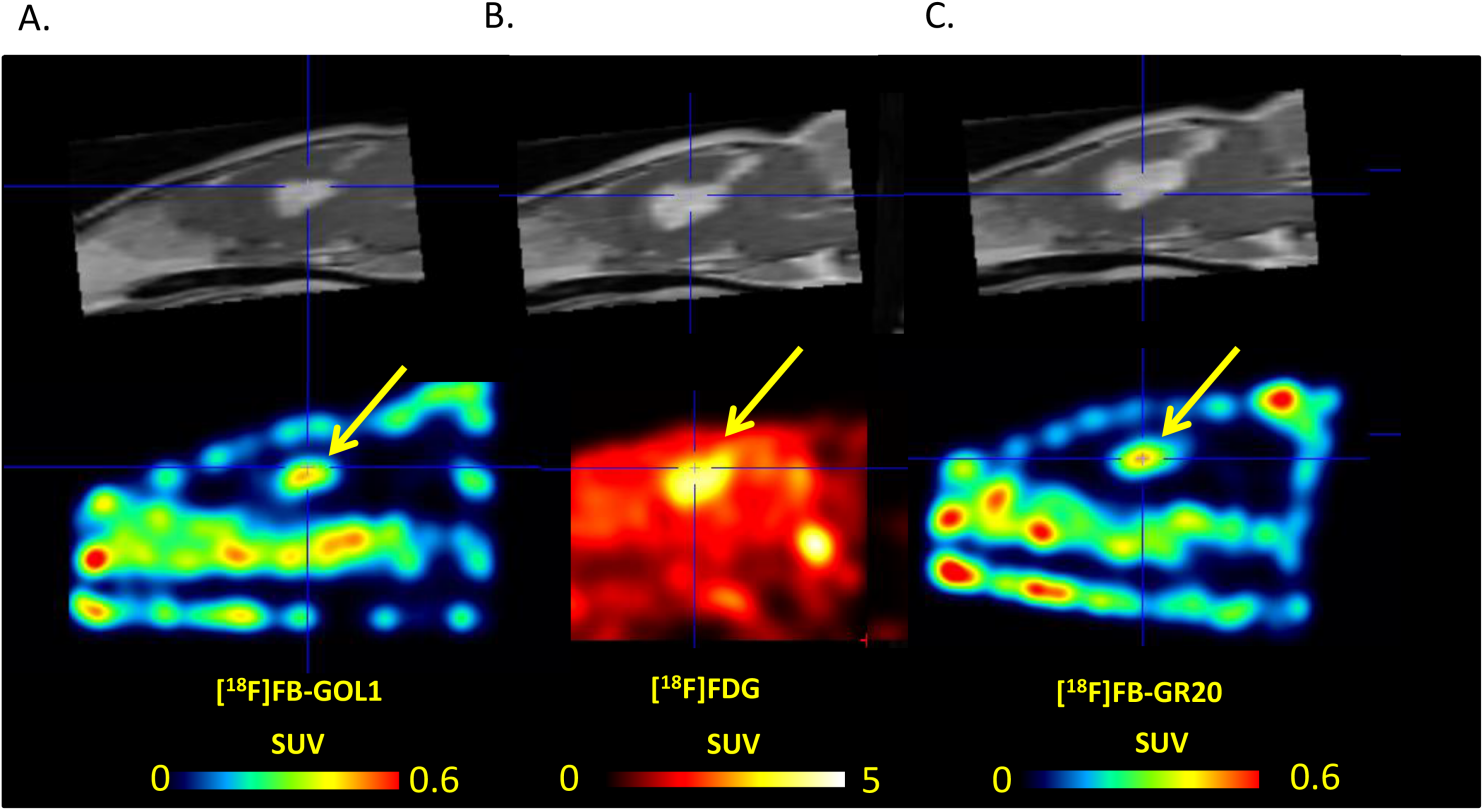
Comparison of tracers uptake (a) [^18^F]FB-Gol1; (b) [^18^F]FDG; (c) [^18^F]FB-GR20. The upper row is T1- MRI with contrast enhancement, the lower row is PET scans. The arrow points to the tumor.
